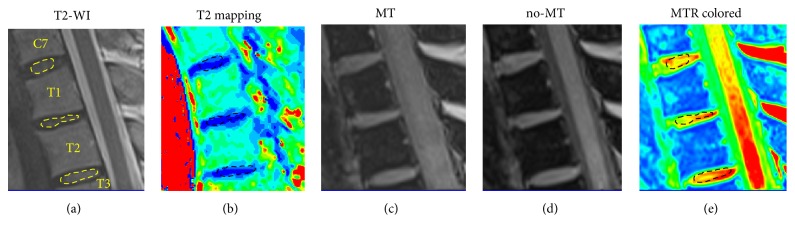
Representative images showing the series of MRI evaluations of the cervicothoracic junction and the placement of ROIs. (a) The T2-weighted MR image of the cervicothoracic junction and the ROIs were manually drawn to include the NP tissue of the discs. (b) T2 mapping image based on the T2 relaxation time value. (c) MTR image that was obtained when the magnetic pulse was applied. (d) No-MT image that was obtained without applying magnetic pulse. (e) MTR colored image according to the MTR values calculated from MT image and no-MT image.
